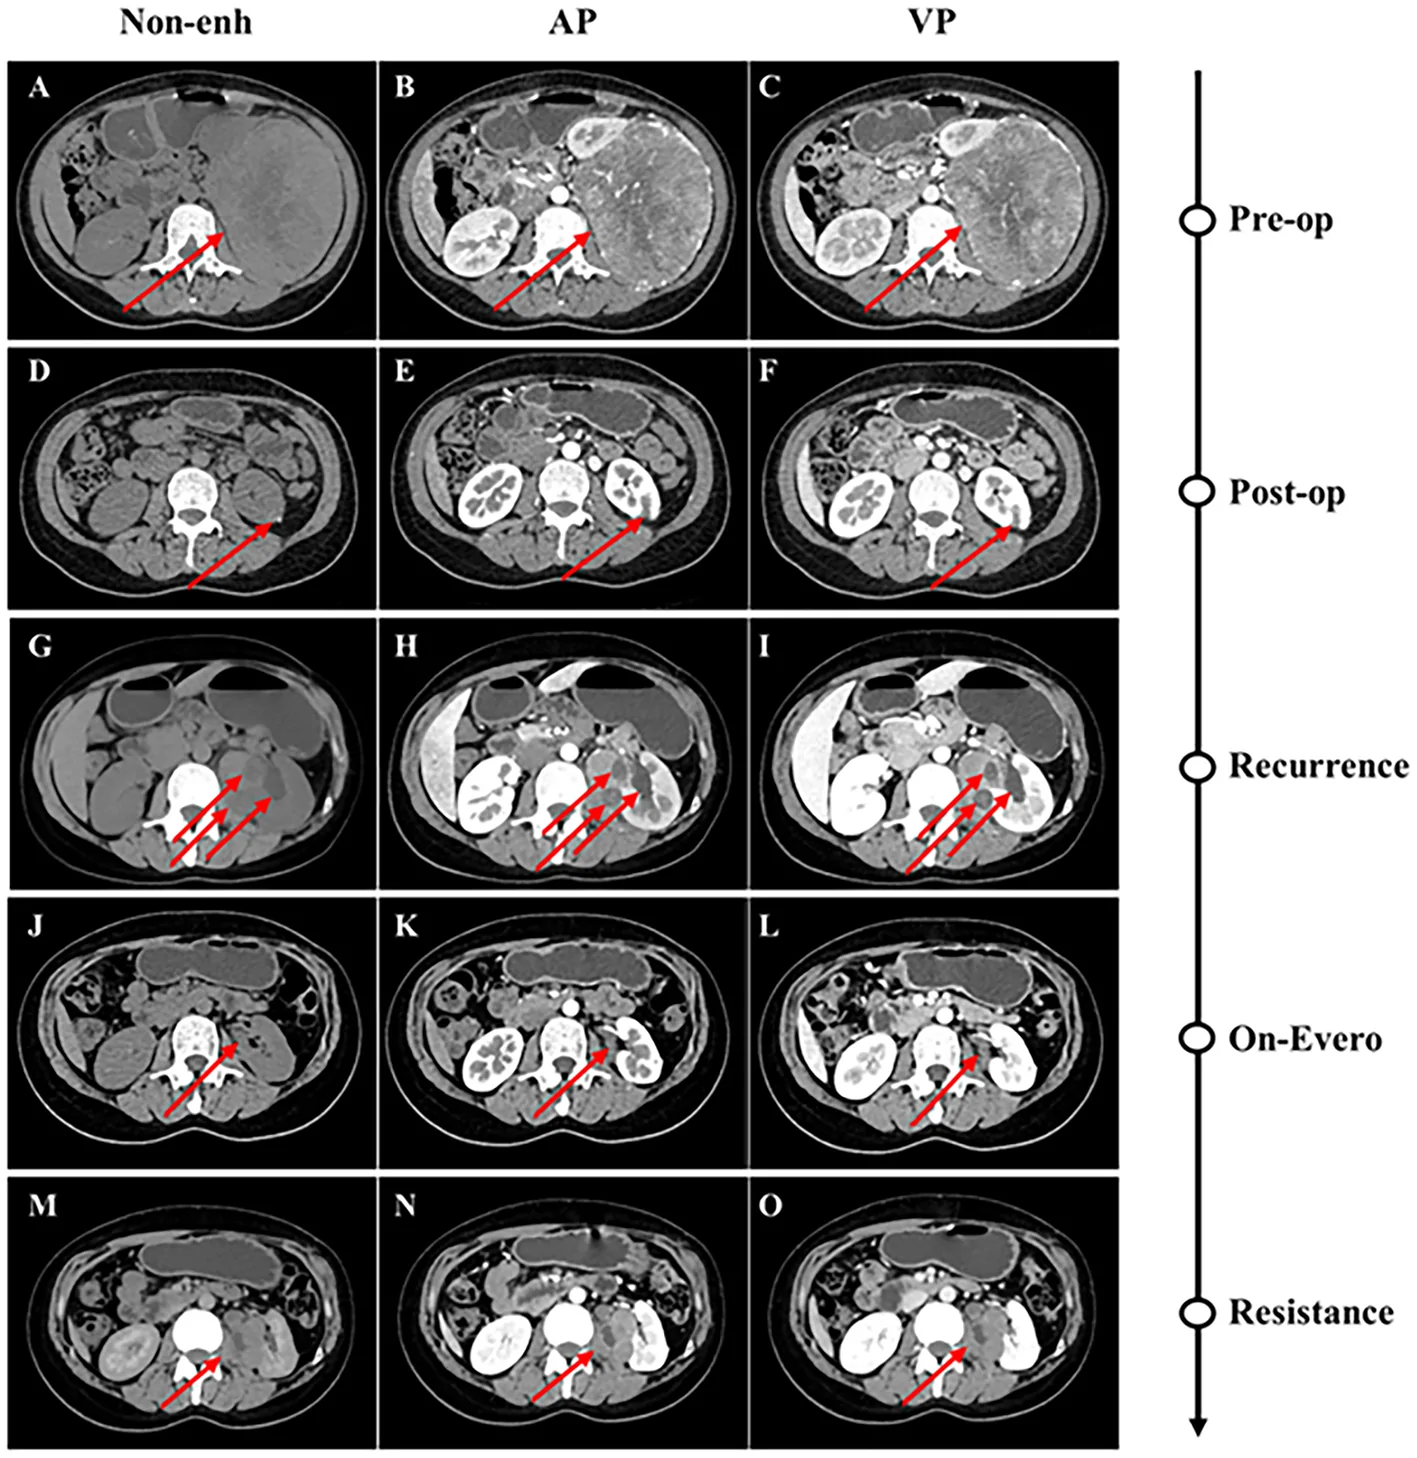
Serial axial contrast-enhanced CT imaging demonstrates the clinical course of metastatic epithelioid angiomyolipoma (EAML). (A–C) Baseline imaging reveals a heterogeneously enhancing left renal mass (arrows), with subsequent CT-guided biopsy confirming EAML. (D–F) Postoperative imaging following left partial nephrectomy shows complete tumor resection without residual disease. (G–I) Surveillance CT at 10 months demonstrates local recurrence in the surgical bed (arrows). (J–L) After initiation of everolimus, follow-up imaging show complete radiographic response. (M–O) Disease progression with new hepatic metastases at 16 months indicates acquired resistance to mTOR inhibition. AP, arterial phase; VP, venous phase; Non-enh, non-enhanced.
